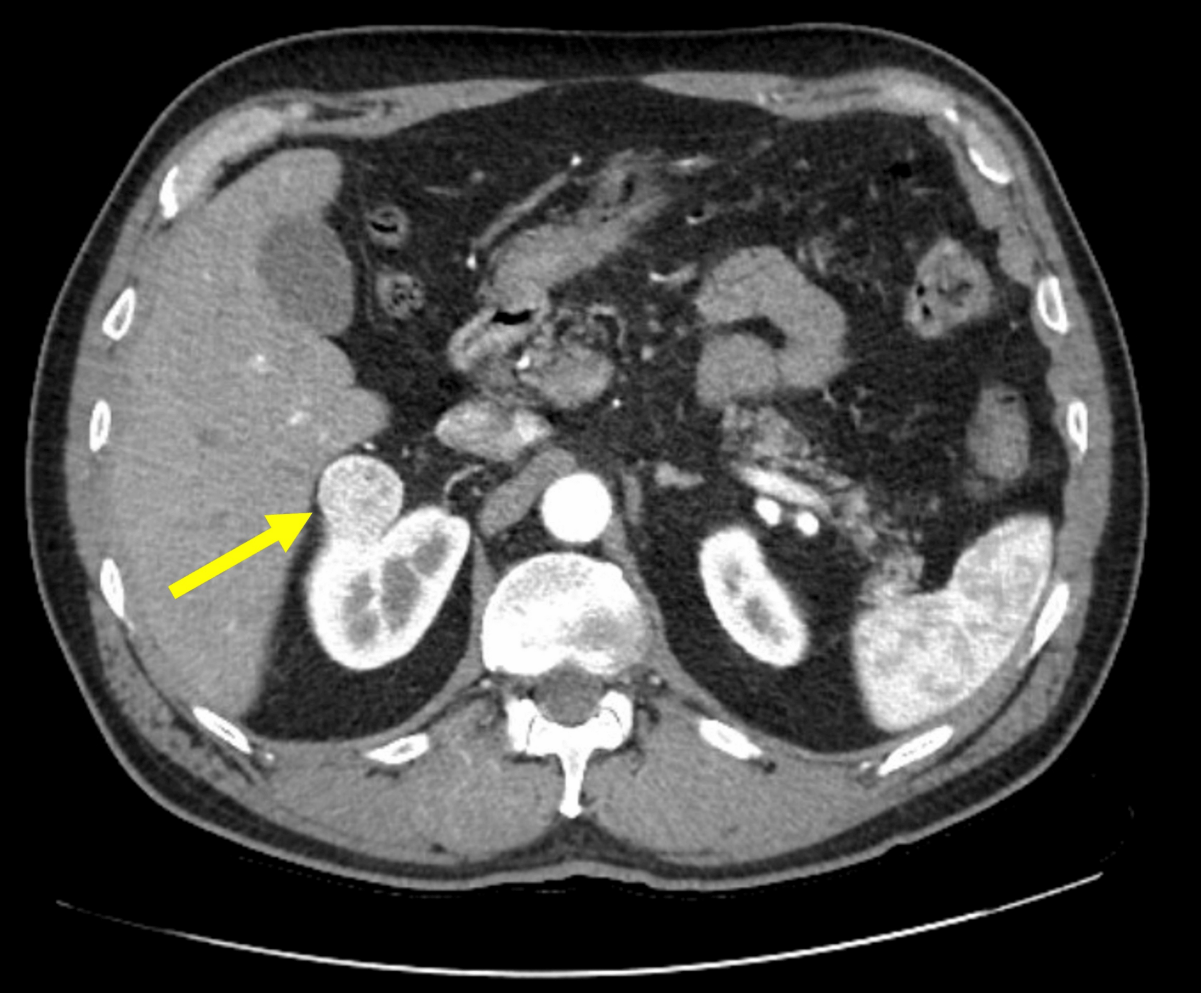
CT abdomen (axial view) demonstrating a 3.1 cm mass in the upper pole of the right kidney (marked by yellow arrow).
